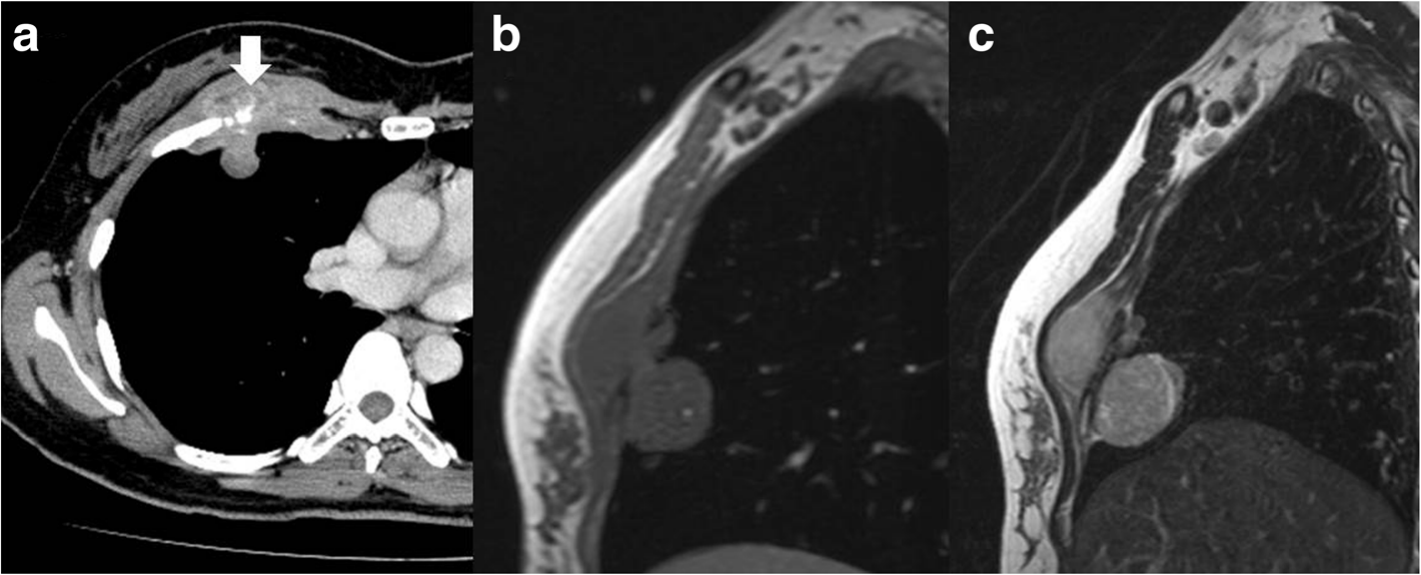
Enhanced chest CT shows a right chest wall tumor with calcification (white arrow) (a). Chest MRI shows an invasive dumbbell-shaped tumor, which is isointense on T1-weighted images (b) and heterogeneously hyperintense on T2-weighted images (c)
